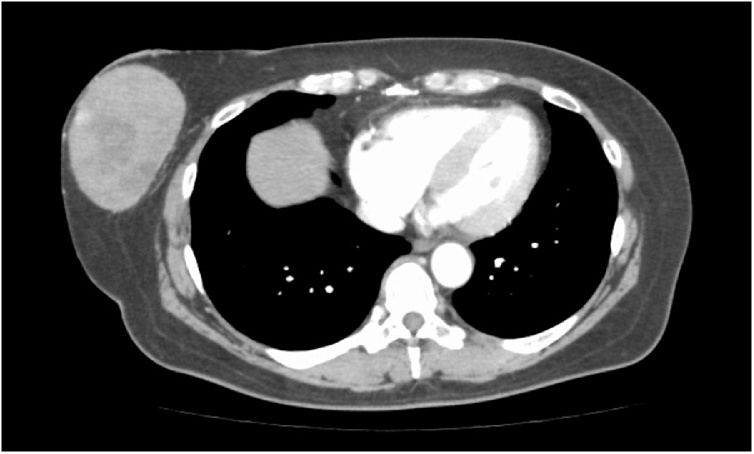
Contrast-enhanced CT showing a cystic mass of 9 cm in diameter in the right breast. The border is clear with accompanying thinning of the skin overlying the tumor.
